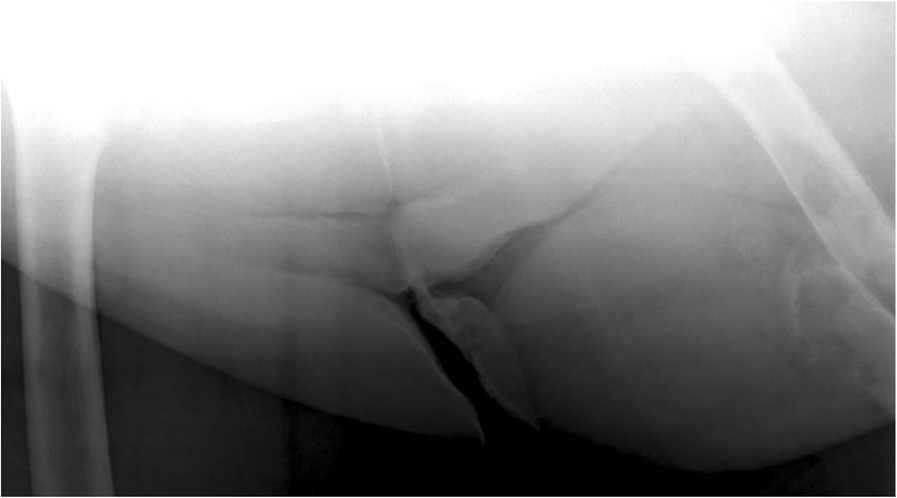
Patient presented with left upper leg pain without history of trauma. The pelvic radiograph showed a displaced fracture of the left femoral shaft with associated lytic lesions
